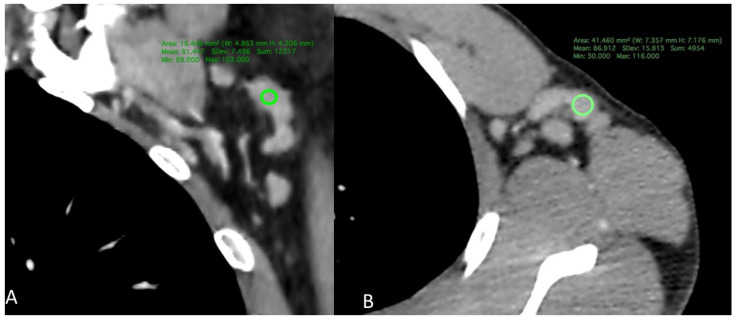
Contrast-enhanced chest CT examples of axillary lymph node cortical density measurements in HIV-positive patients. (A) CD4 count > 500 cells/μL; (B) CD4 count < 200 cells/μL.
